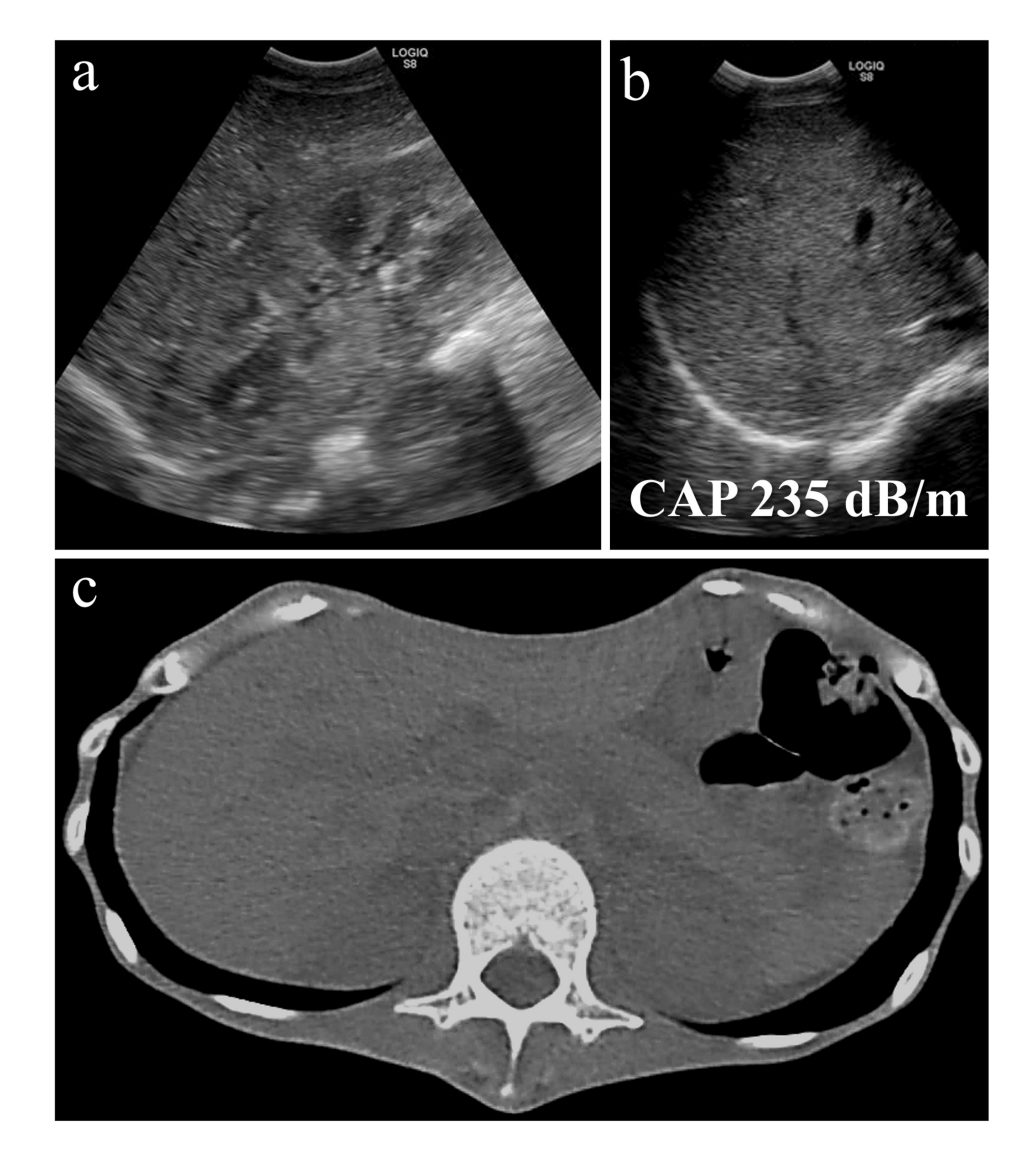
Abdominal imaging during the phase of rapidly elevated transaminases CAP: controlled attenuation parameter Abdominal imaging during the phase of rapidly elevated transaminases. (a) Abdominal ultrasonography did not show hepatorenal echo contrast. (b) Deep echo attenuation was not observed. (c) Abdominal computed tomography did not show hepatomegaly
